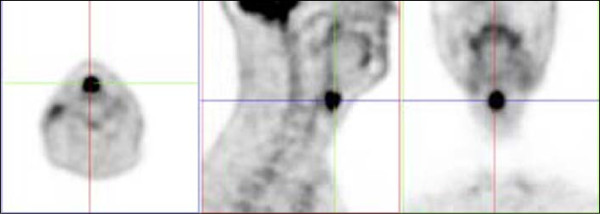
Case example of a false positive FDG-PET finding. Axial, sagittal and coronal views of the PET scan are displayed and demonstrate an uptake in the supraglottic region. The area was free of tumor when examined through careful panendoscopy and multiple deep directed biopsies were negative for neoplasia.
